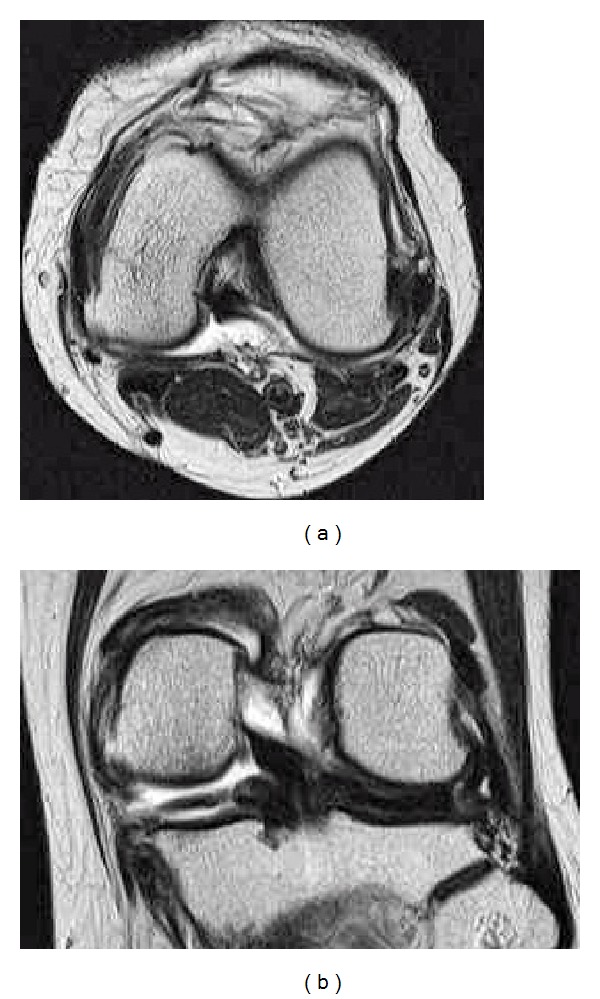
T2 weighted axial (a) and coronal (b) MR images taken 9 months postoperatively demonstrate complete disappearance of the medial meniscal cyst.
